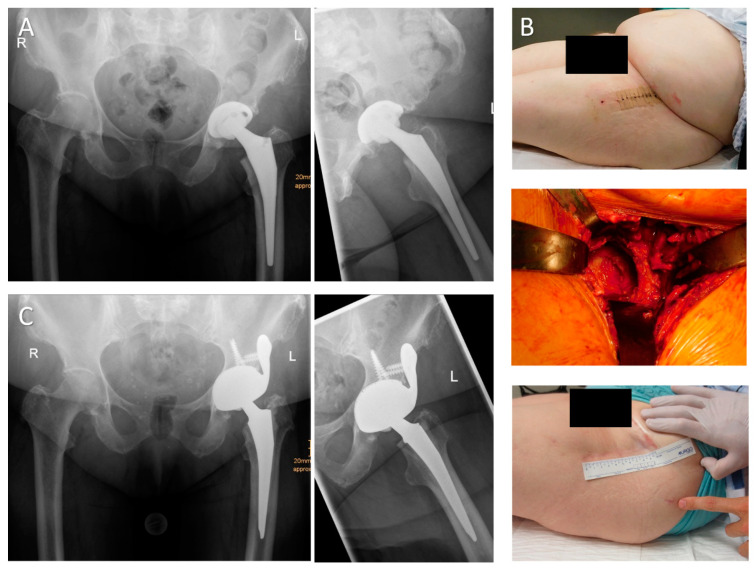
(A) A.p. and axial radiograph of an 80-year-old female patient presenting with transverse periprosthetic fracture and medial protrusion of the cup two weeks after cementless hip arthroplasty. (B) The patient suffered from multiple co-morbidities and her BMI (body mass index) was 39 kg/m^2^. After removal of the loose acetabular and femoral implant via the DAA, the acetabular fracture line was visible. To prevent iatrogenic lesions of the TFL during stem extraction, a “tensor-snip” was performed. The screw fixation through the implant’s iliac flange was performed by insertion of the screws via the DAA and subsequent fixation with a long screwdriver introduced via a gluteal stab incision in order to avoid any proximal extension of the approach. (C) Radiographic imaging (a.p. and axial view) 18 months postoperatively show a well-fixed acetabular implant (HHS: nine preoperatively, 64 postoperatively; OT 126 min).
